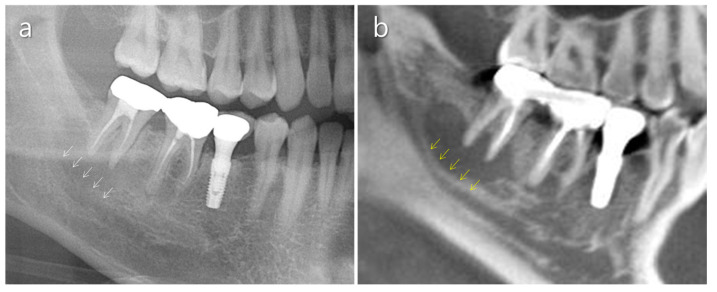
Usefulness of CBCT for identifying the mandibular canal. (a) Unclear mandibular canal border in a conventional panoramic radiograph. Periapical bone resorption adjacent to the mandibular canal makes identification of the upper border of the canal difficult (white arrows). (b) A CBCT scan shows the upper border of the mandibular canal remarkably well (yellow arrows).
